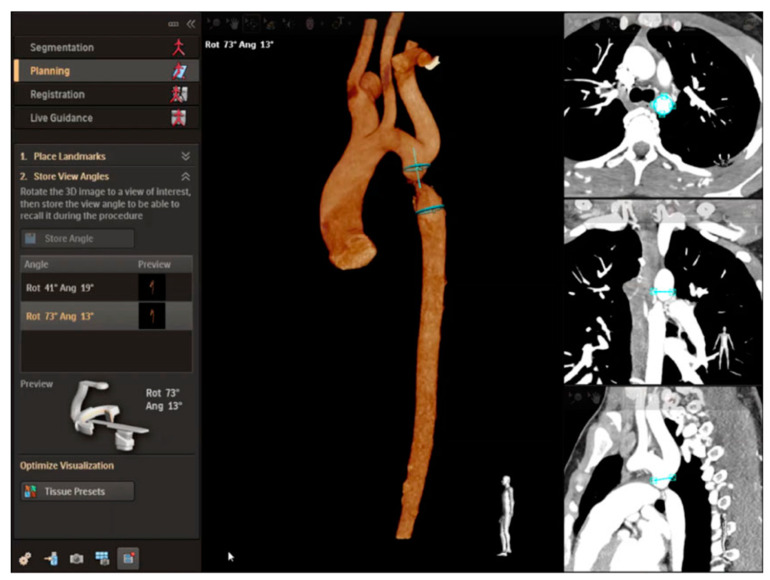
Overlay planning using the VesselNavigator system (Philips Healthcare, Best, The Netherlands) for a patient with a discrete coarctation of the aorta (CoA) prior to stenting using CT images. The area of interest is outlined in blue. Revised from ref [9].
